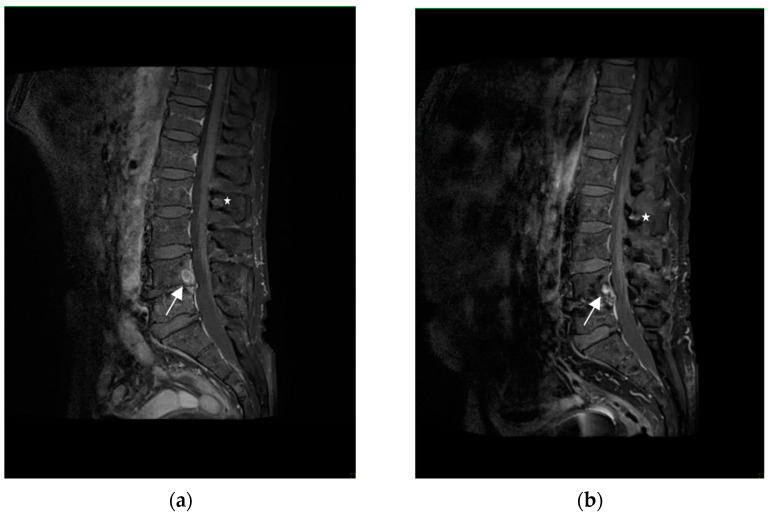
T1-weighted images with fat saturation and with gadolinium contrast administration, sagittal plane. Contrast enhanced lesion in L4 vertebral body ((a)—white arrow) has reduced its size ((b)—white arrow). Similarly, the lesion in the spinous process L2 ((a)—white asterisk) has decreased in size ((b)—white asterisk).
